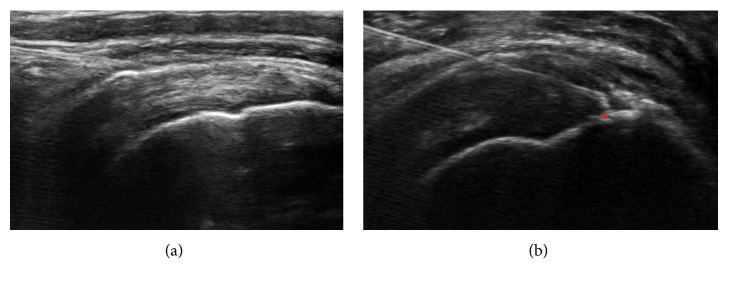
(a) Ultrasound-guided injection into (a) the subacromial bursa and (b) the supraspinatus tendon. ^∗^The tear site of the supraspinatus tendon.
